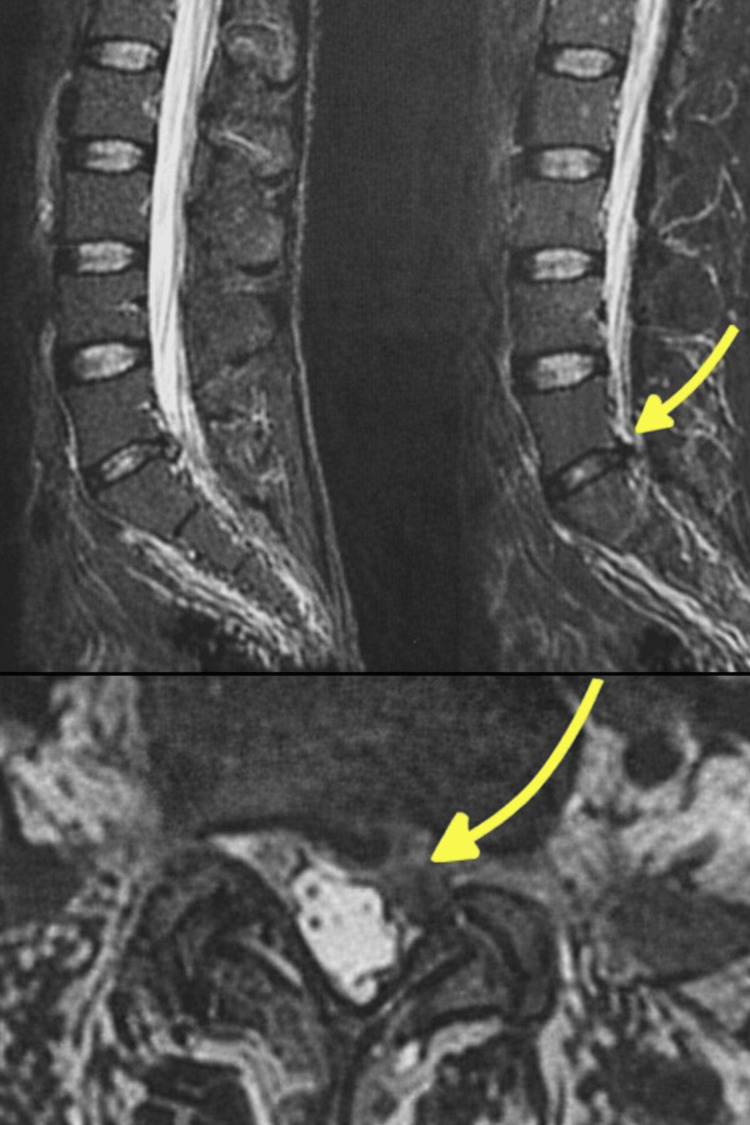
A sagittal and axial view of a lumbar MRI showing a large L5–S1 disc herniation.
